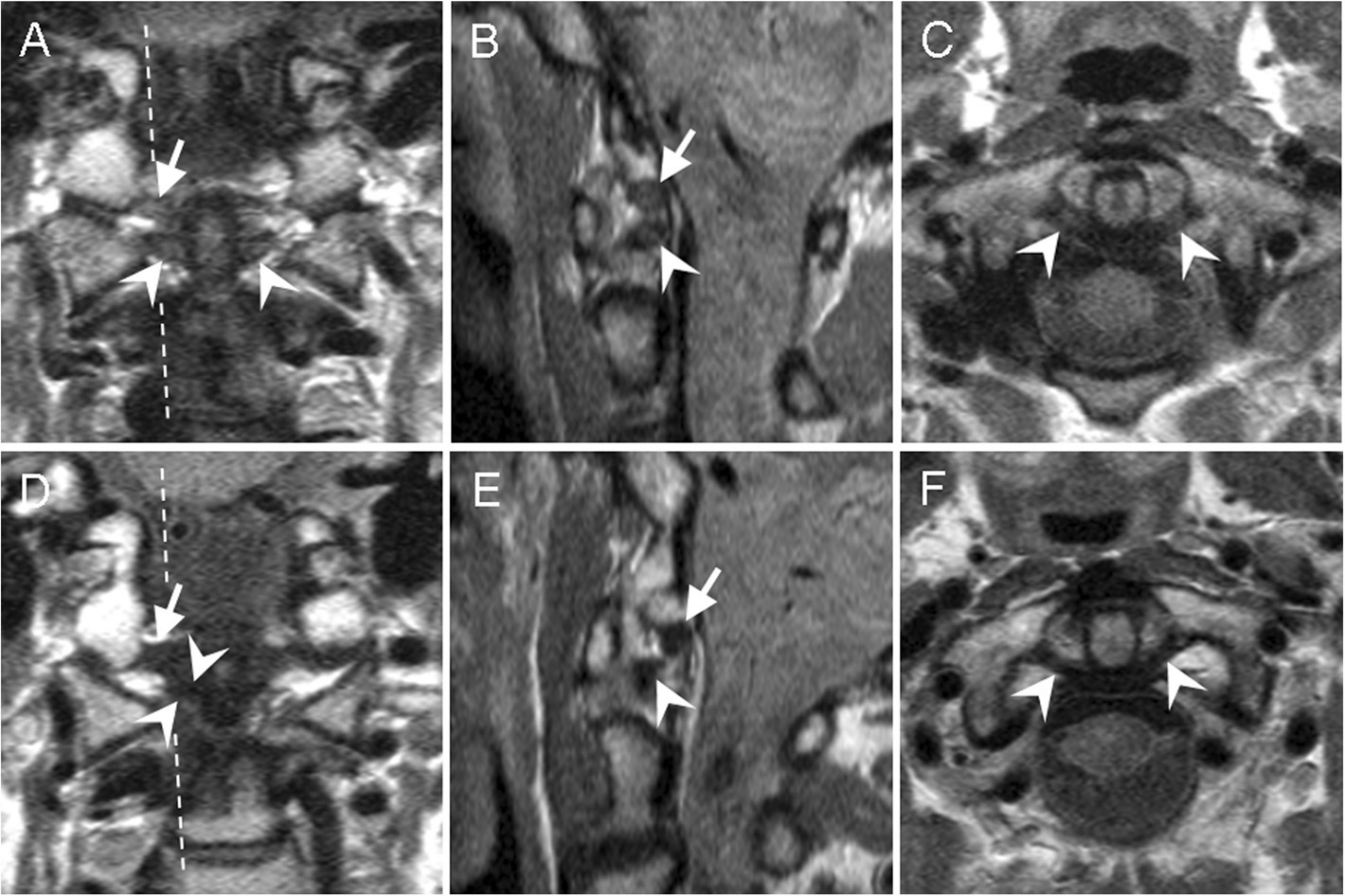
Scoring high signal intensities of alar and transverse ligaments on upper neck MRIs. Proton-density-weighted, fast-spin echo, 1.5 Tesla MRI sections were performed in (A, D) coronal, (B, E) sagittal, and (C, F) axial directions. MRIs were from two healthy women, aged (A-C) 44 years old, and (D-F) 60 years old. Broken lines mark the sagittal plane. (A-C) The transverse ligament is indicated with arrow heads. The high intensity signal was scored 2 by reader A, 1 by reader B, and 2 by consensus; in the second evaluation, the same signal was scored 2 by both readers independently. The alar ligament is indicated with arrows. (A, B) The high intensity signal was graded 2 by both readers independently; in the second evaluation, the same signal was scored 2 by reader A, 3 by reader B, and 2 by consensus. (D-F) The transverse ligament (arrow heads) and alar ligament (arrows) were scored 0 by both readers independently in both evaluations.
